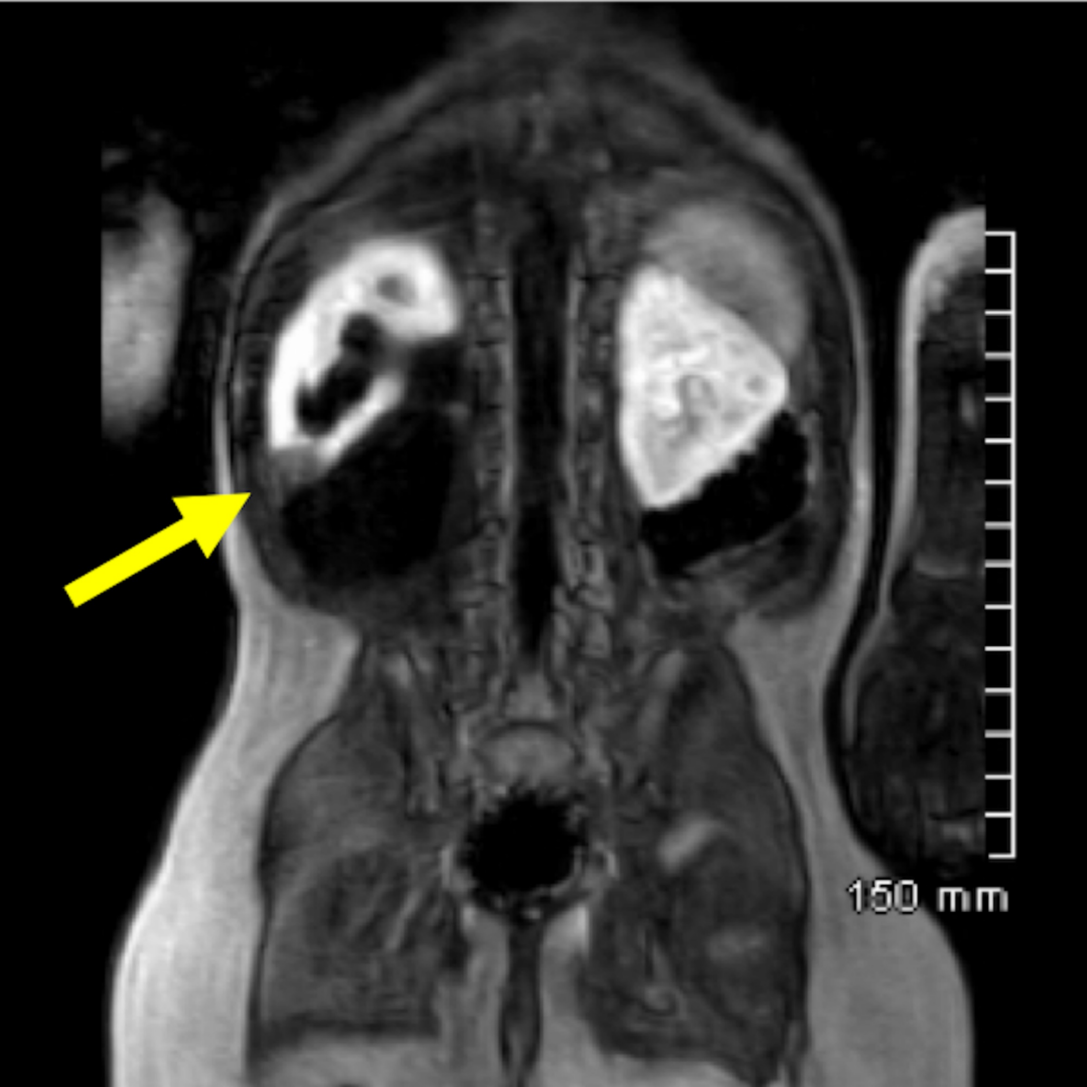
Magnetic resonance urogram Dynamic T1-weighted post contrast coronal image of magnetic resonance urogram. The yellow arrow shows scarring in the lower pole of the right kidney.
